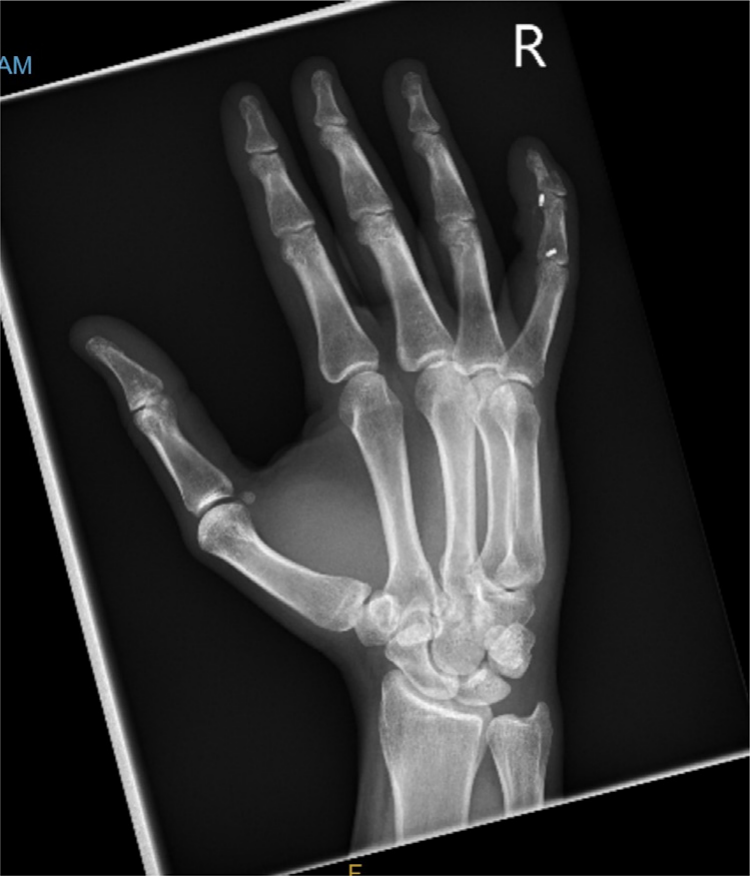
Oblique X-Ray of right hand (Case 1).
